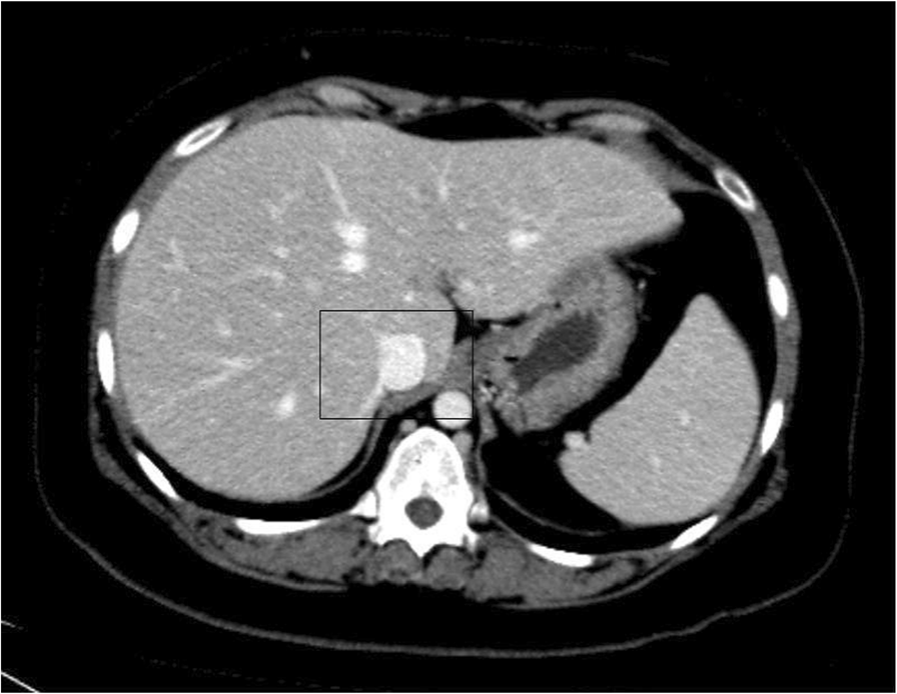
Computed tomography scan showing a normal intra-hepatic portion of the inferior vena cava (boxed region).
